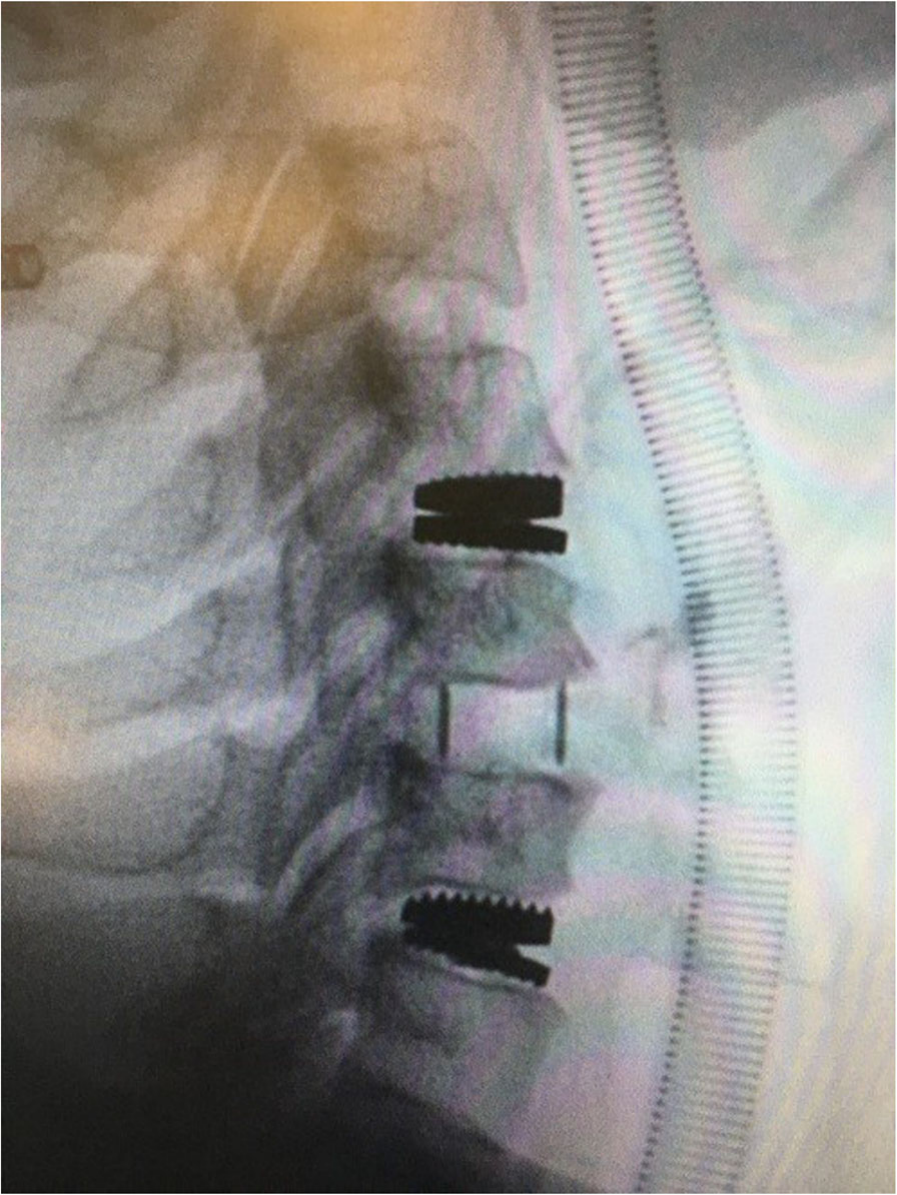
Plain radiograph after hybrid construction using cervical disc prosthesis for C4–C5 and C6–C7, and cervical cage for C5–C6
